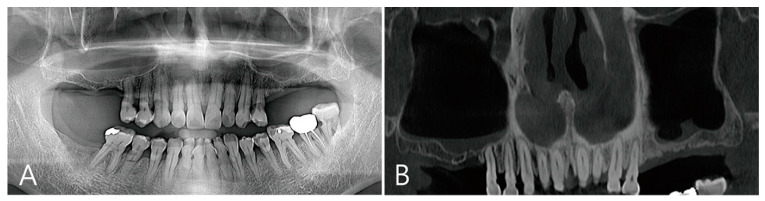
Case 2. (A) On preoperative panoramic radiography, severely atrophied residual ridges were observed in the missing area of the left and right maxillary molars, and the maxillary sinuses were pneumatized; (B) a panoramic image of the preoperative CBCT showed slight thickening of the sinus membrane on the sinus floor.
